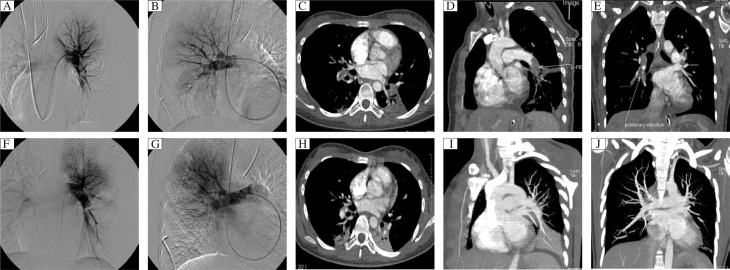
14- year-old female with dyspnea for 4 d and hypotension for 1 d. A and B: Supraselective angiogram shows multiple emboli. First investigator scored arterial obstruction as 16 and perfusion as 7; Miller index was 67%. Second investigator scored obstruction and perfusion as 16 and 13, respectively; Miller index was 85%. C-E: Axial transverse and reconstruction CT scan shows occlusive clots in right middle lobar artery and bilateral inferior lobar pulmonary artery (arrow). First investigator calculated Qanadli index as 50%; second investigator scored as 50%. F and G: After catheter fragmentation, supra-selective angiogram was performed in the real time of therapy; the restoration of blood flow in left pulmonary artery improved obviously; however, in right pulmonary vasculature the blood flow did not improved immediately. H-J: Fifteen days after therapy, the patient did CTPA as a follow-up evaluation. The endoluminal filling defects in bilateral lung almost disappeared. Doctor A scored Qanadli index as 5%; Doctor B scored as 2.5%.
